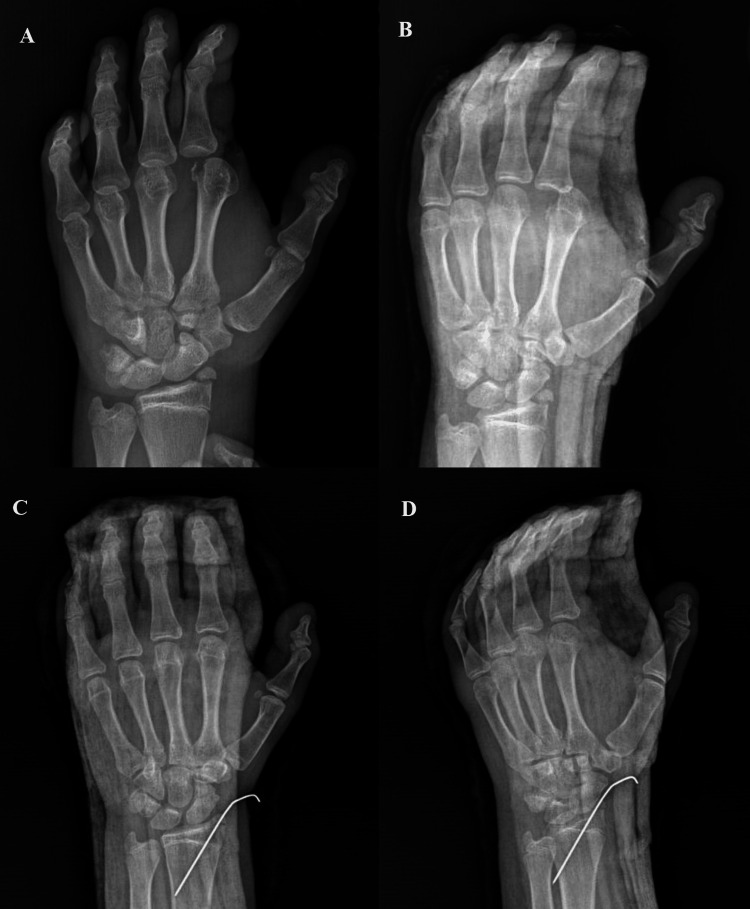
Preoperative (A, B) and postoperative (C, D) radiographic images show the dorsal dislocation of the left index metacarpophalangeal joint and concomitant radial styloid fracture
